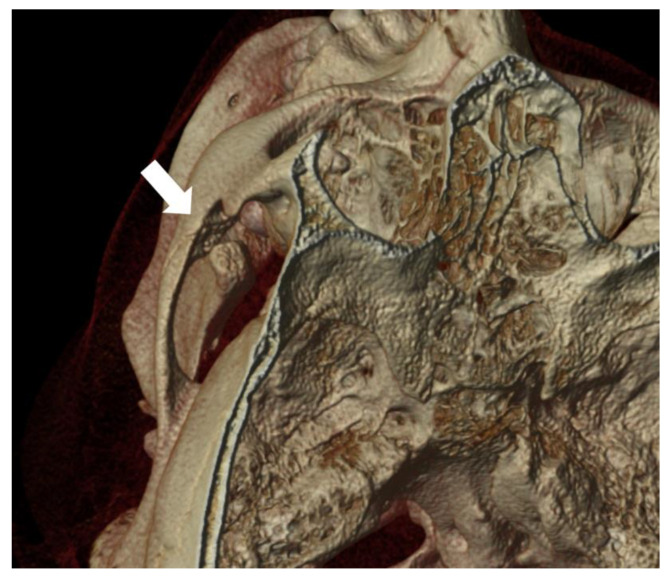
Three-dimensional reconstruction of computed tomography scan in a patient with Jacob disease. The white arrow shows the pseudojoint formation between the zygomatic bone and the elongated coronoid process of the mandible.
